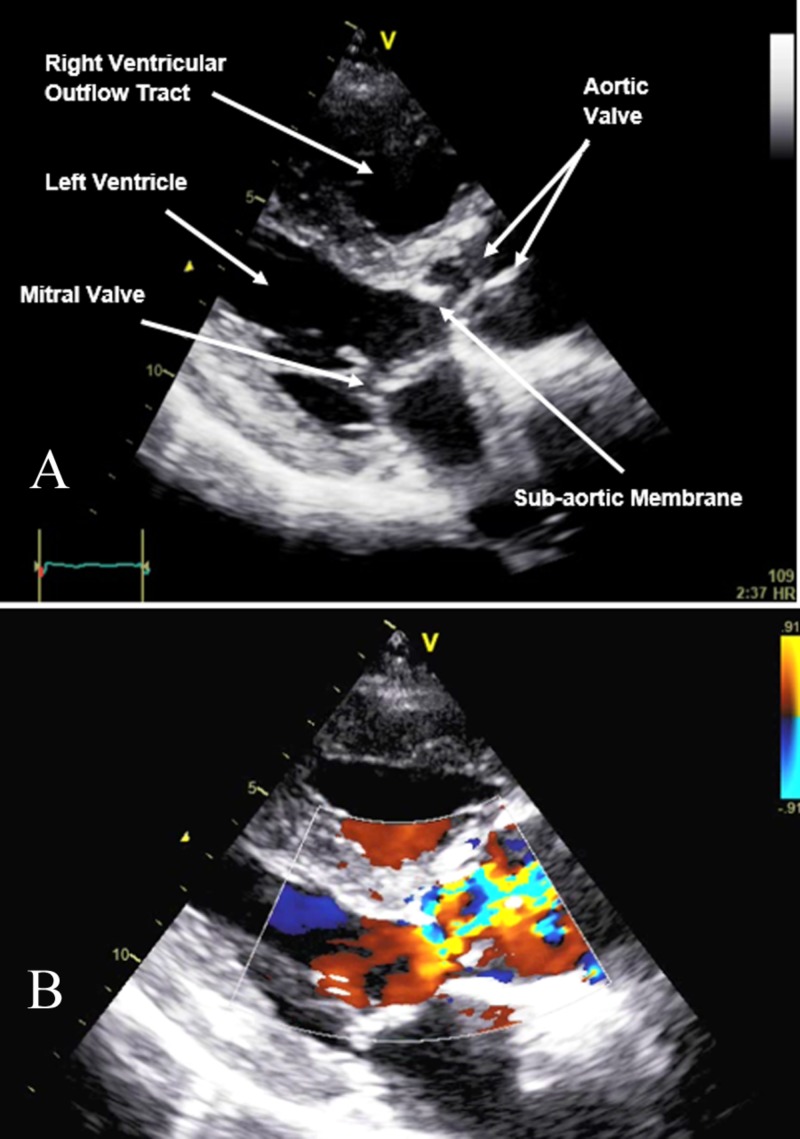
Transthoracic Echocardiogram A: Parasternal long axis of transthoracic echocardiogram showing subaortic membrane. Other normal structures seen are aortic valve, mitral valve, left ventricle and right ventricular outflow tract. B: Parasternal long axis of transthoracic echocardiogram with Color Doppler applied at the level of the aortic valve revealing turbulence below the level of the aortic valve.
